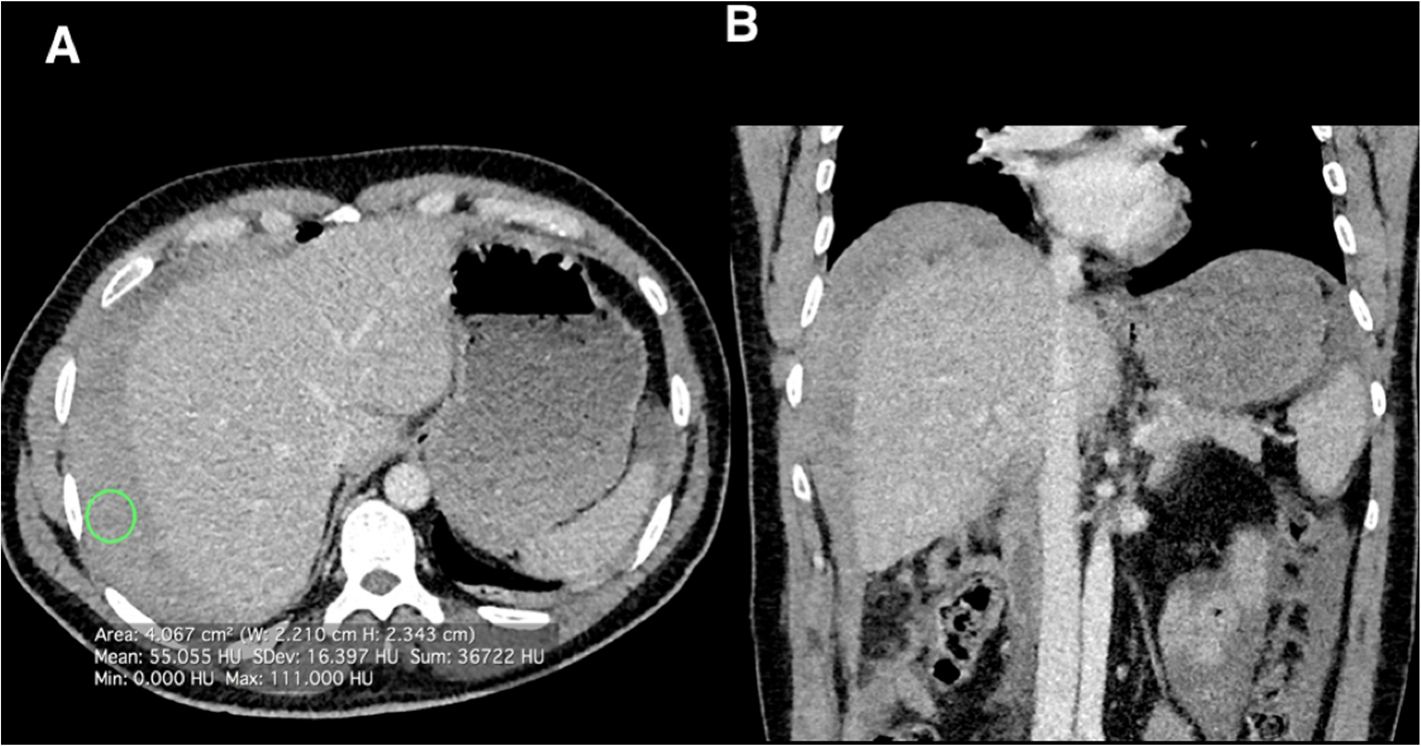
Axial and coronal contrast enhanced CT of the abdomen in a patient several hours post-cholecystectomy. There is large volume perihepatic fluid with an average Hounsfield unit of 55 consistent with post-cholecystectomy bleeding
